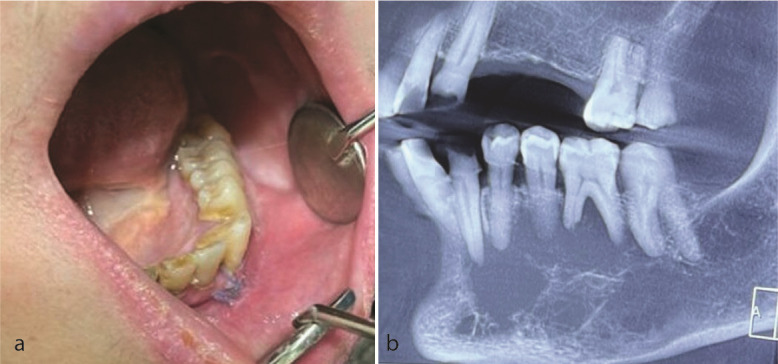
a: Photograph shows mild buco-lingual expansion of left mandible, b: Cone beam computed tomography (CBCT) displays a large lytic radiolucent lesion with right-angle septa
